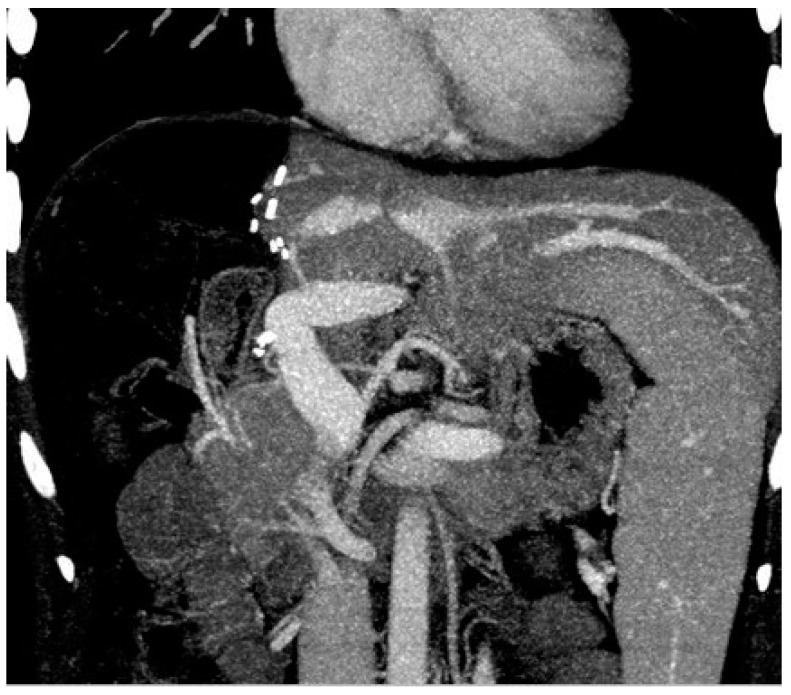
The coronal reformatted computed tomography imaging shows that the portal vein reconstructed with the elbow patch technique is patent.
